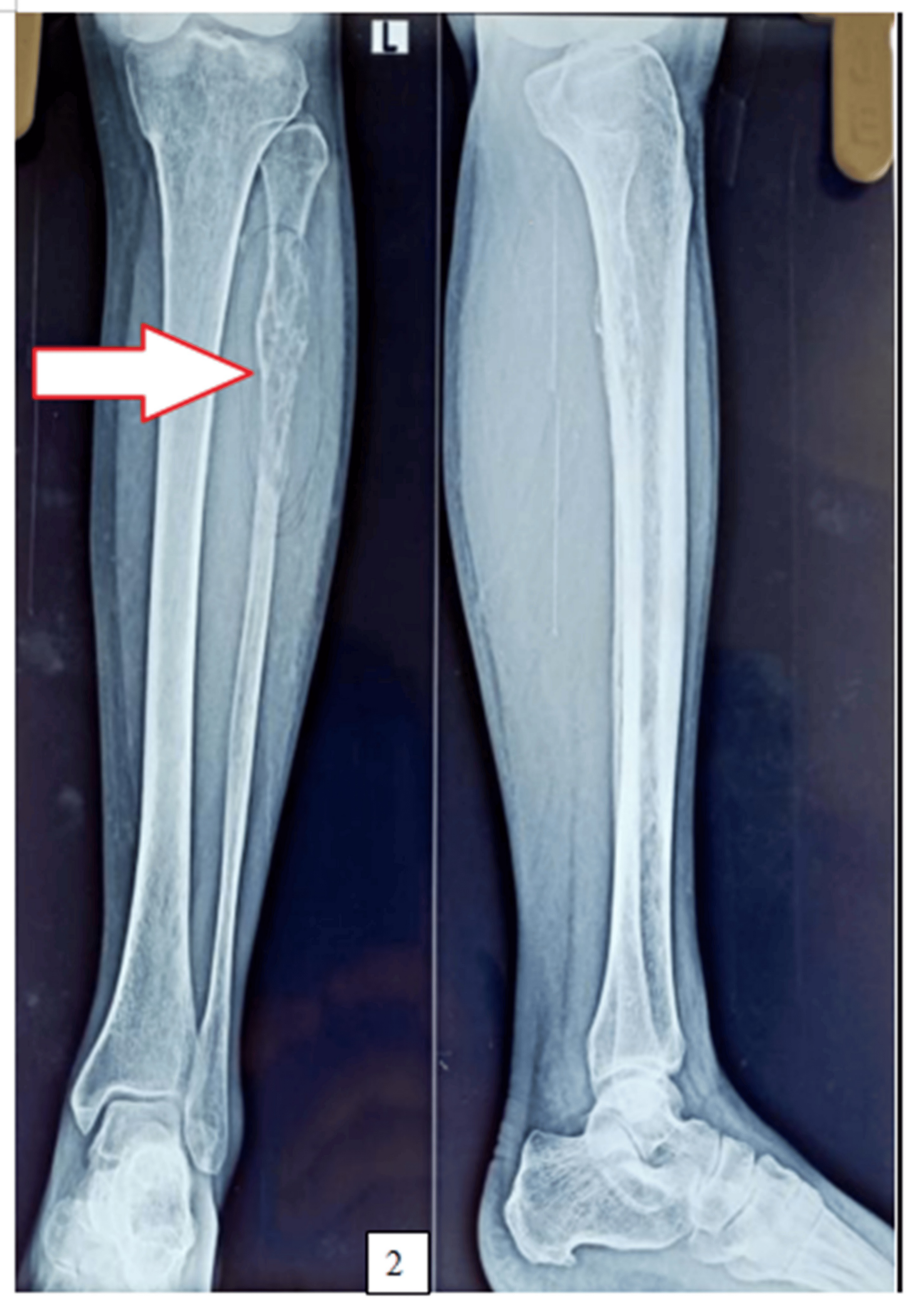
Radiograph: X-ray anteroposterior and lateral view of the left leg shows an expansile lytic lesion involving the proximal shaft of the fibula (marked with a white arrow)
